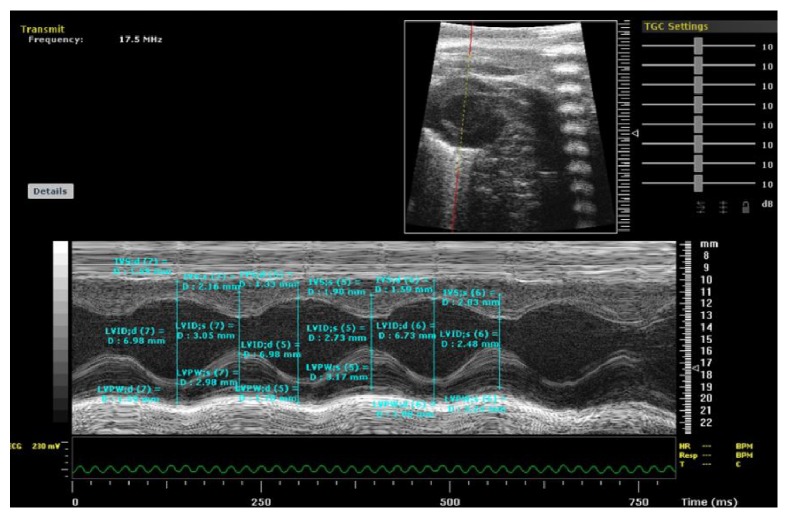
Representative tracing of echocardiography: LV function.
